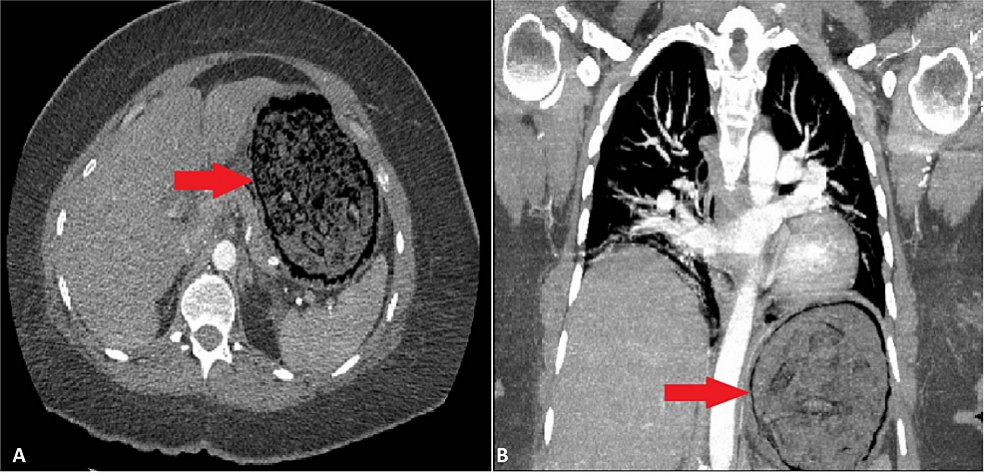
CT scan demonstrating air in the gastric wall (red arrow) on axial (A) and coronal (B) views.
